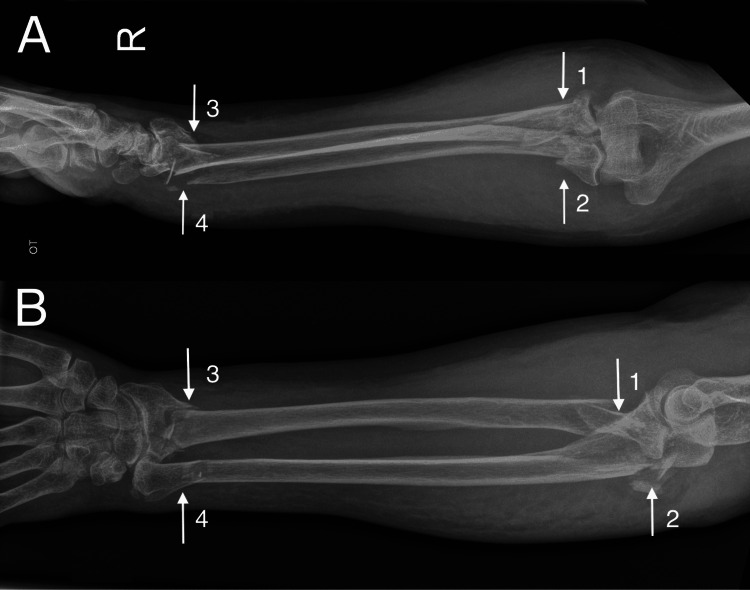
A: lateral radiograph of right forearm; B: anteroposterior radiograph of right forearm Arrow 1: Radial head fracture; Arrow 2: Olecranon fracture; Arrow 3: Distal radius fracture; Arrow 4: Distal ulna fracture
